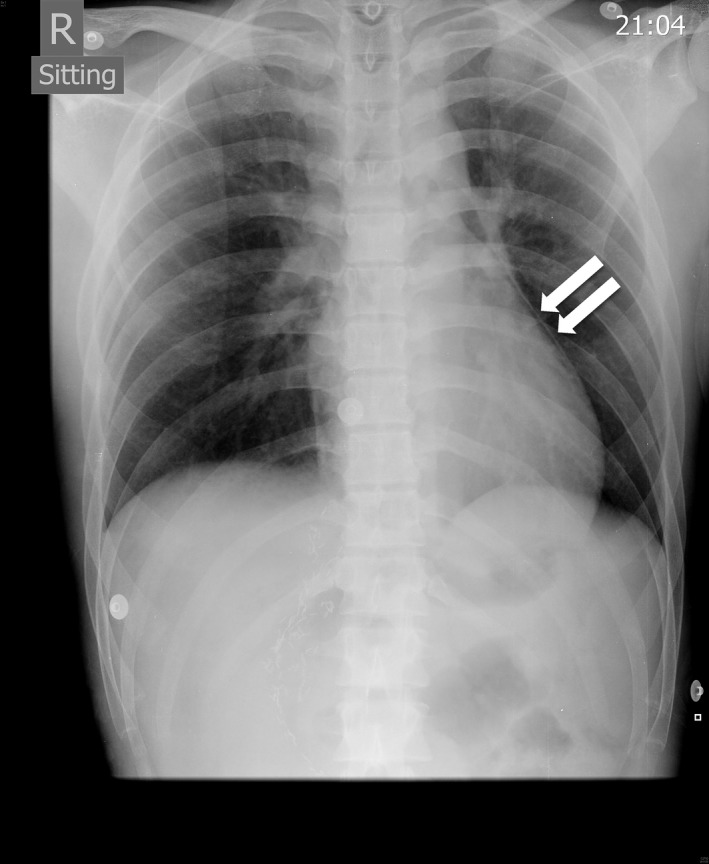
Chest radiograph (arrows indicate a radiolucency behind the heart).
